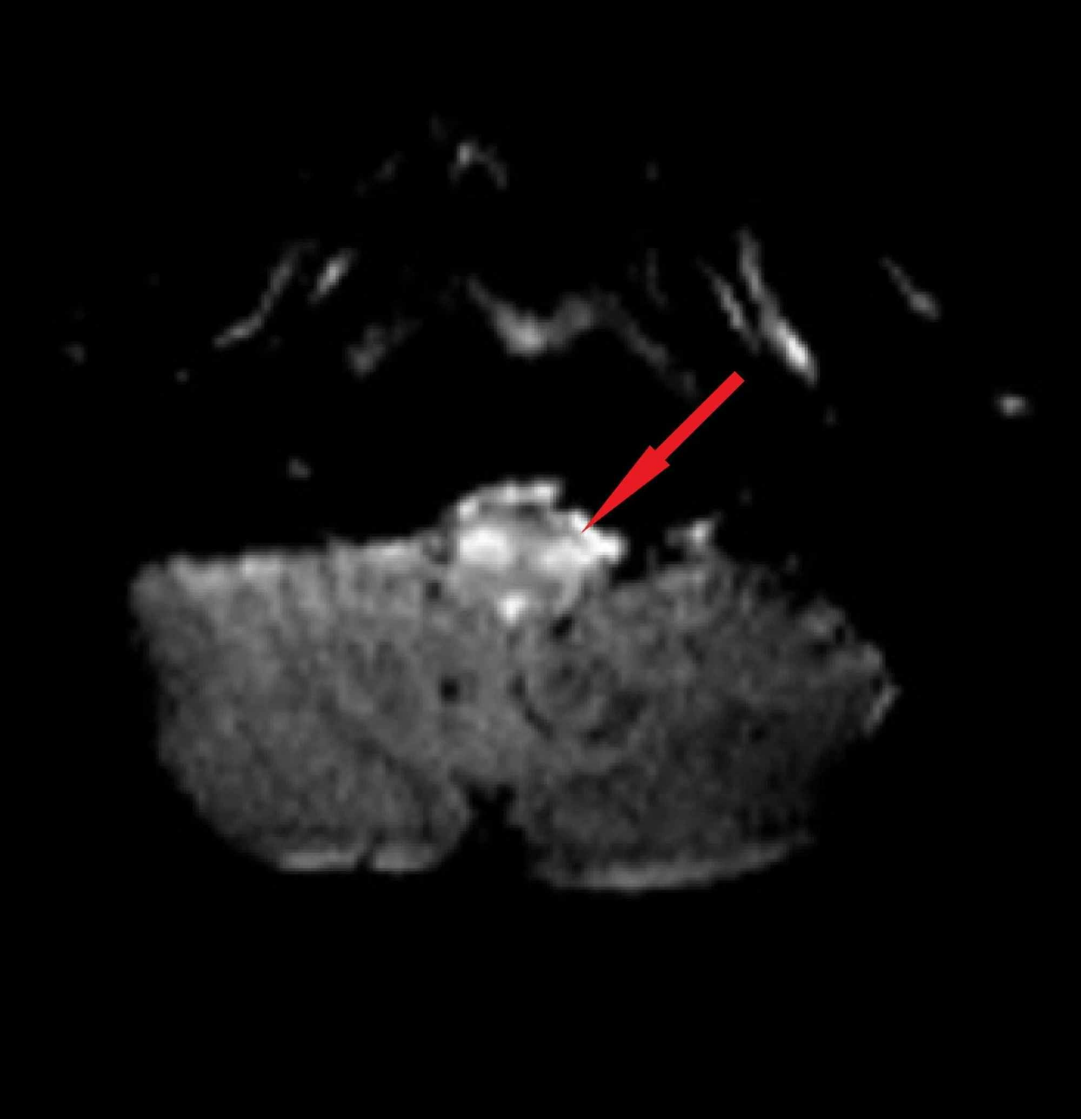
Hyperintensities in the Inferior Olivary Nuclei on Axial Fluid-Attenuated Inversion Recovery Magnetic Resonance Imaging
